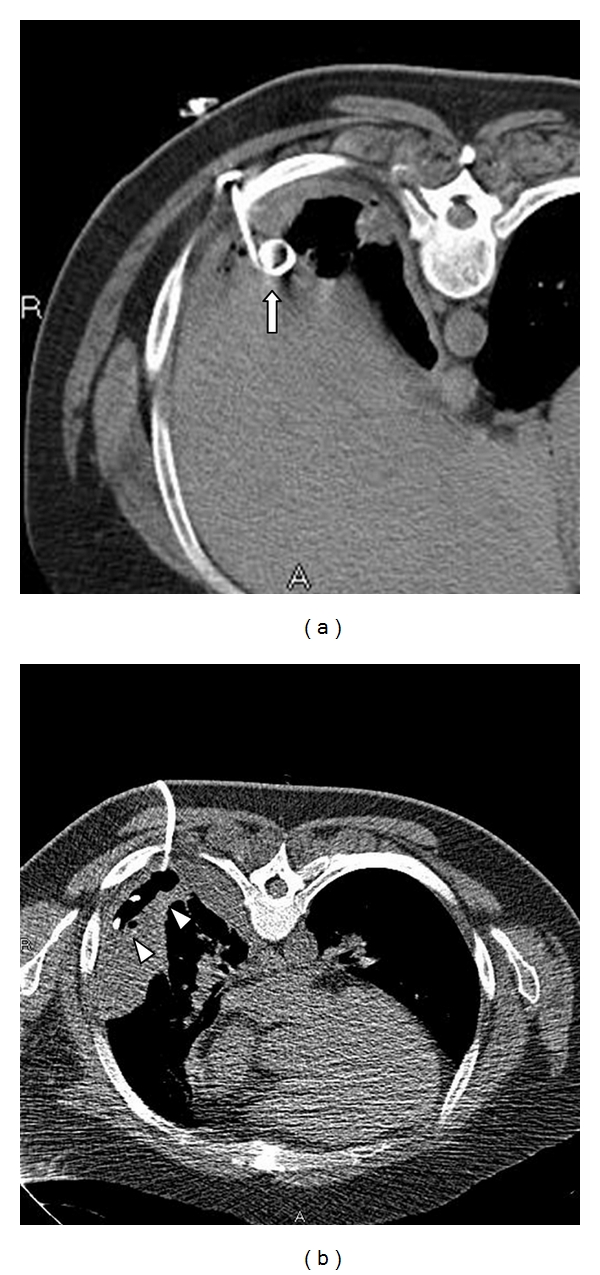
CT images after chest tube drainage. (a) Image shows placement of pigtail catheter (arrow) in the posterior recess confirmed with CT. (b) Placement of small-bore pigtail catheter (arrowheads) in the small loculated effusion with the help of CT guidance.
